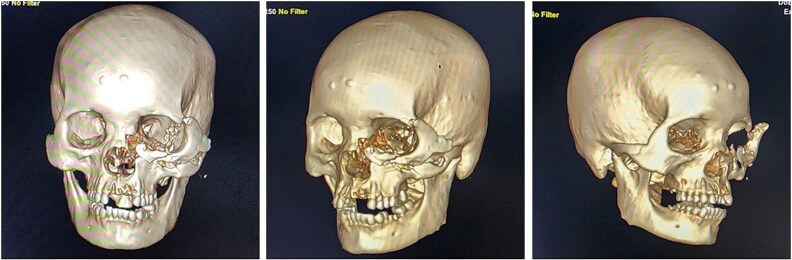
CT scan findings of the patient after a bear mauling.
